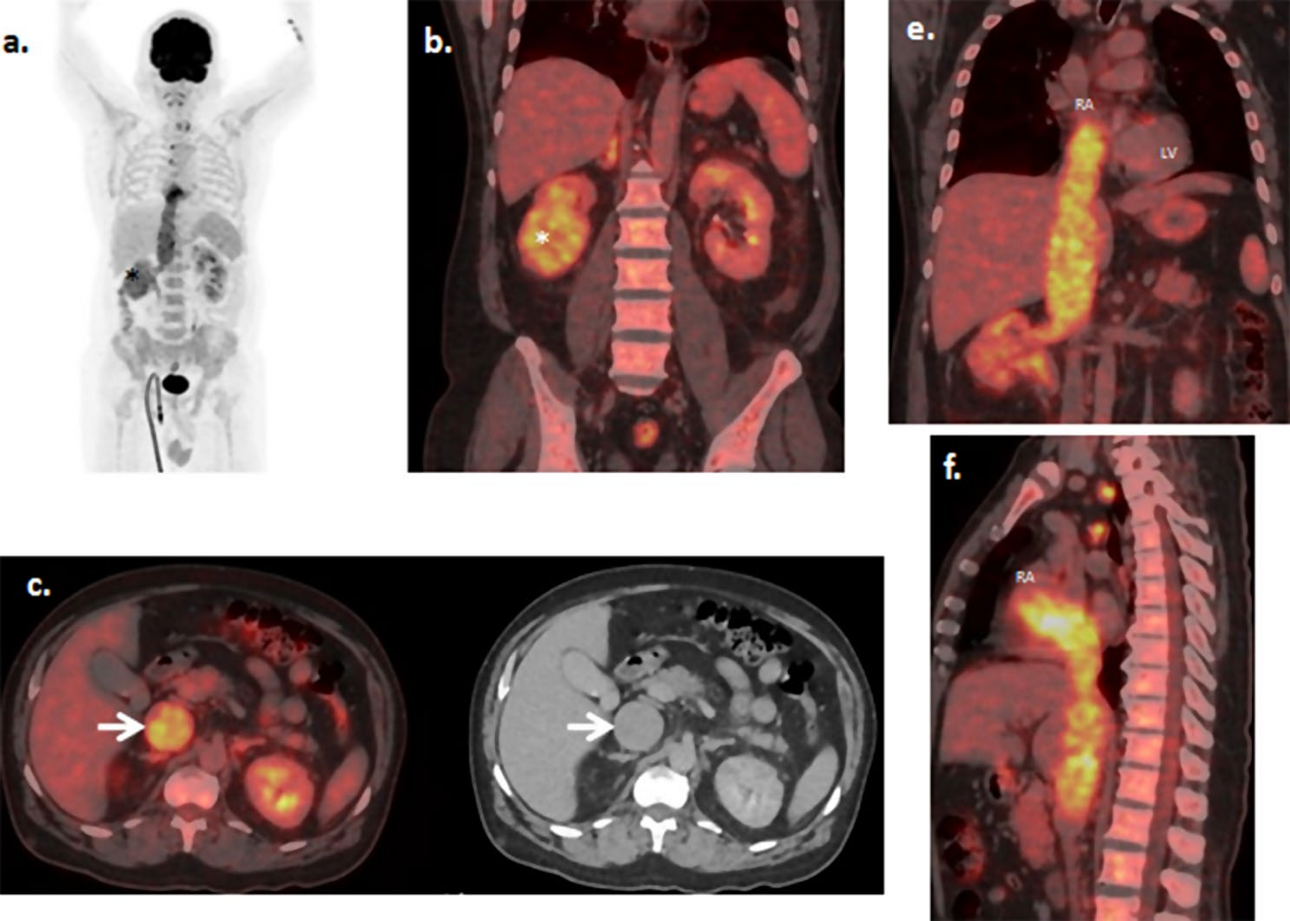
A 49 year gentleman, recent diagnosed case of right renal carcinoma, underwent PET/CT for staging by intravenous injection of 7mCi of F-18 FDG. Maximum Intensity Projection image (MIP, a) and sagittal fused PET/CT image (b) show metabolically active right renal mass in its lower pole (*asterisk*). Fused and CT axial images (c) show diffuse intravascular FDG uptake in right renal vein and dilated IVC suggestive of tumor thrombus (*white arrows*). The fused coronal (e) and sagittal (f) images show the tumor thrombus reaching upto right atrium
